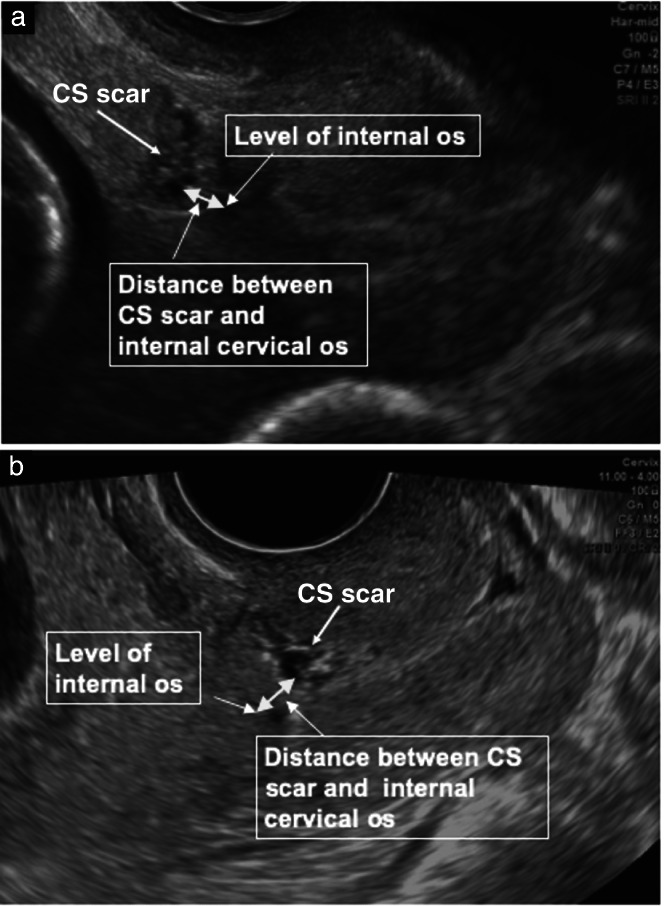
Grayscale ultrasound images showing measurement of the distance from the Cesarean section (CS) scar to the internal cervical os in the sagittal plane in a case with a CS scar above the cervix (a) and a case with a CS scar in the cervix (b). The level of the internal os was determined using color flow mapping of the uterine artery.
